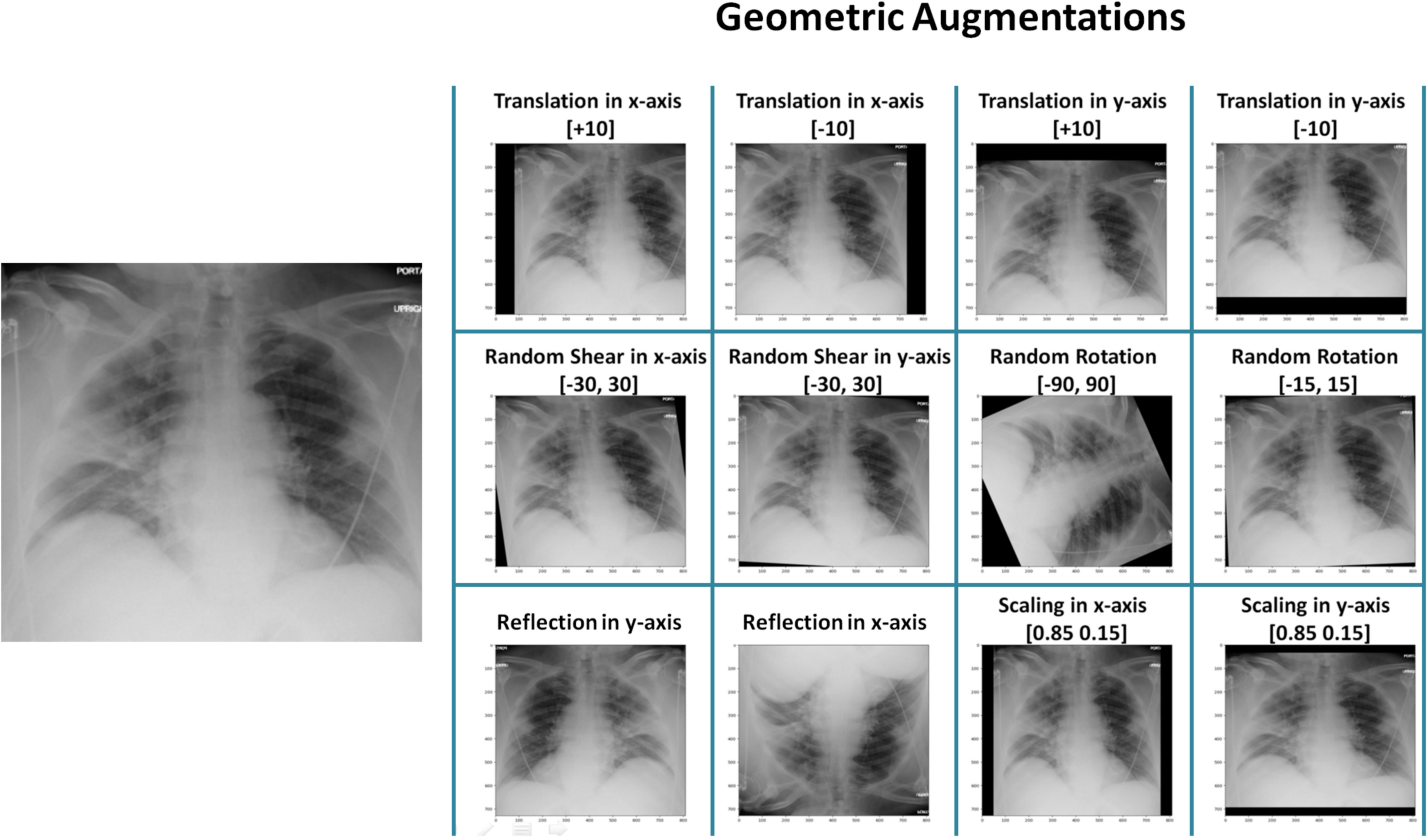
Application of different geometric augmentation transformations to a COVID-19 X-ray image.
